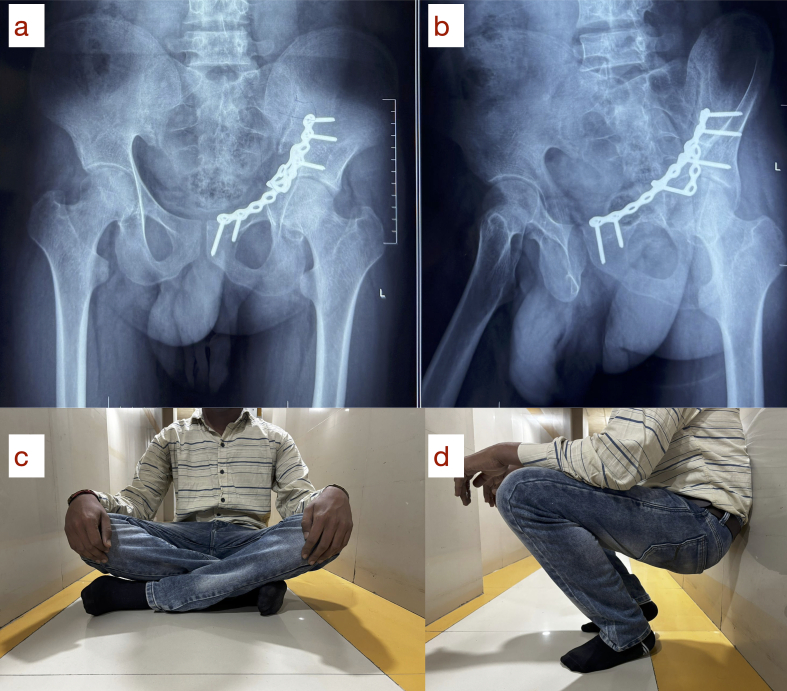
One-year follow-up radiographs demonstrating complete fracture union: (a) anteroposterior view and (b) obturator oblique view. Corresponding clinical photographs showing full functional recovery with the ability to (c) sit cross-legged and (d) squat.
